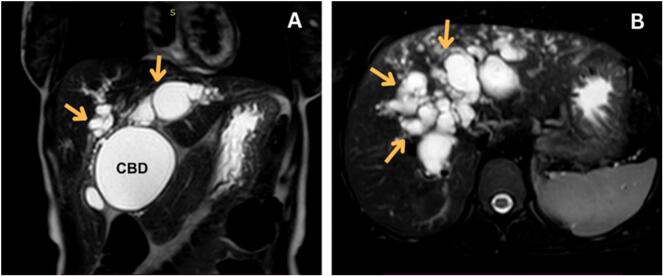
Coronal (A) and axial (B) T2-weighted MRI images demonstrating dilated intrahepatic and extrahepatic biliary ducts (arrows), with marked dilation of the common bile duct (CBD).
